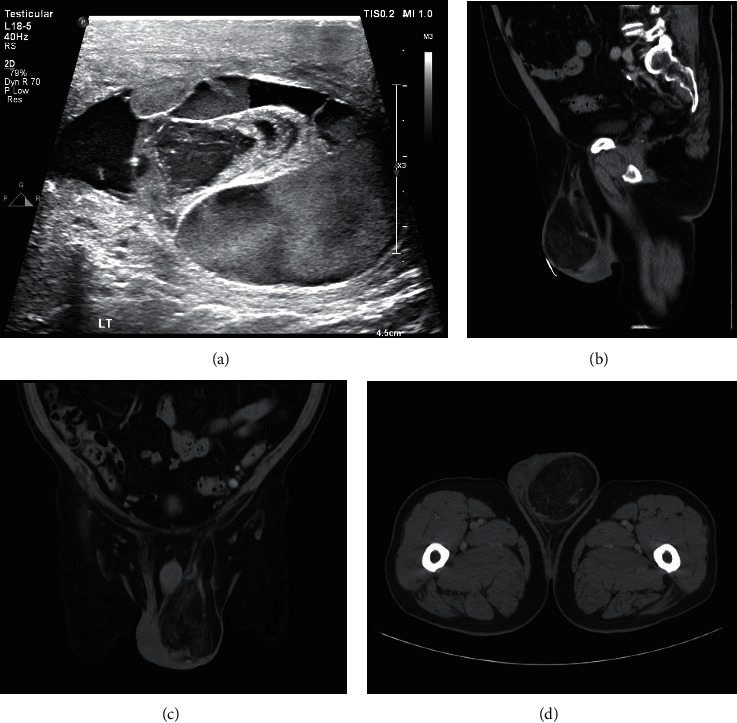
Case example: preoperative imaging comparison of ultrasound and CT scan. The images are of a 72-year-old male who presented with a firm testicular mass with associated scrotal swelling. (a) A scrotal ultrasound shows a mixed echotexture heterogeneous lesion within the left scrotum. Unfortunately, sonographic evaluation of inguinal or scrotal lesions lacks specificity in distinguishing fat- or bowel-containing inguinal hernia from a soft tissue sarcoma, such as a liposarcoma. (b–d) Sagittal, coronal, and axial contrast-enhanced preoperative CT images in the same patient demonstrate a predominately low-attenuation fatty 10 cm mass lesion within the inferior left inguinal canal and left scrotum. The presence of a thickened capsule around the periphery of the lesion as well as prominent enhancing internal septations with nodularity increases suspicion of a well-differentiated liposarcoma.
